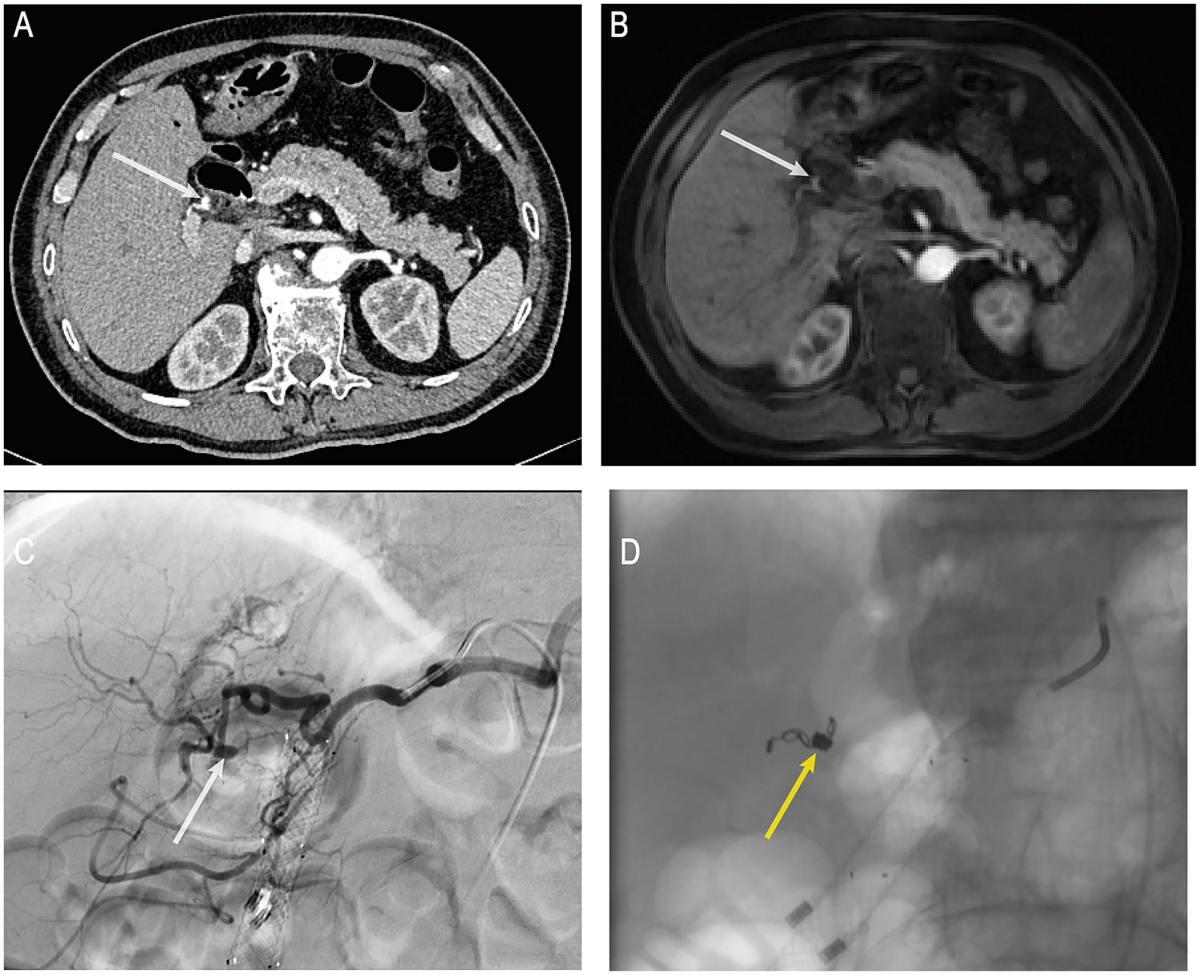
(A) Post-hemorrhage hepatic artery CTA. (B) Pre-hemorrhage enhanced MRCP. (C) Post-hemorrhage hepatic artery DSA. (D) Post-TAE under DSA guidance. CTA, computed tomography angiography; MRCP, magnetic resonance cholangiopancreatography; DSA, digital subtraction angiography; TAE, transcatheter arterial embolization. White arrows indicate the location of the pseudoaneurysm in the right hepatic artery; yellow arrows indicate the site of arterial embolization.
